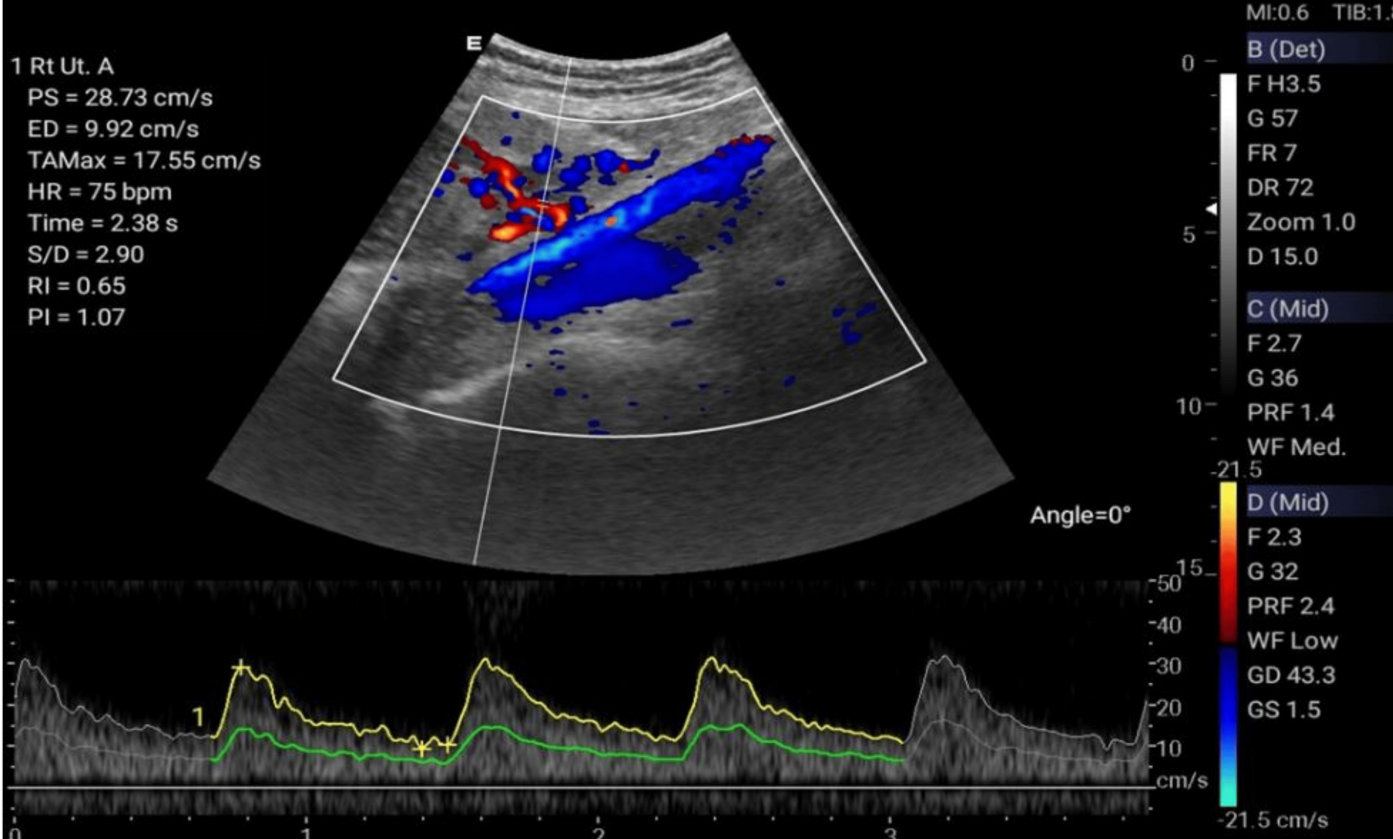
Right uterine artery Doppler.
